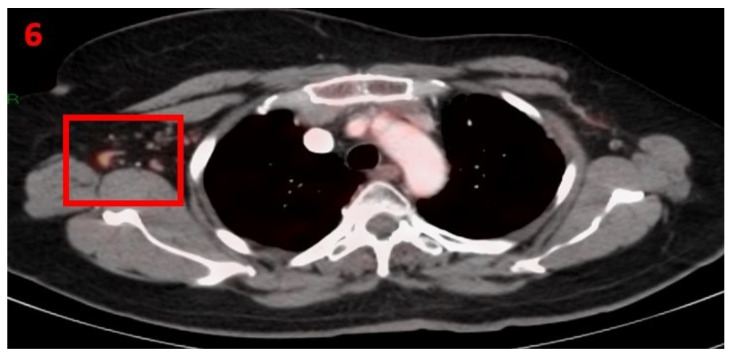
[18F]FDG PET-CT showing hypermetabolic uptake in the 6th patient with lymphadenopathy in the right axillary region (red square).
